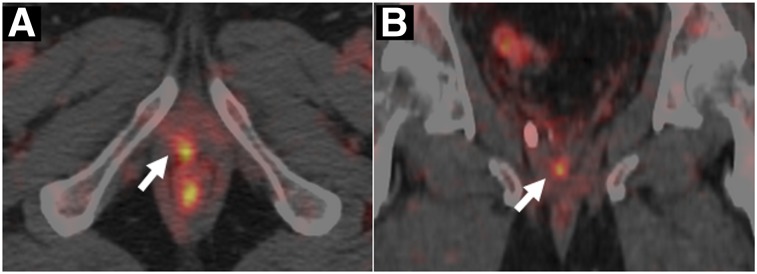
Axial (A) and coronal (B) PET/CT images of pelvic area in prostate cancer patient (Gleason grade 4 + 3) 60 min after injection of 200– 250 MBq of ^64^CuCl_2_, demonstrating tracer uptake in foci of local relapse (arrow). (Reprinted with permission of (24).)
